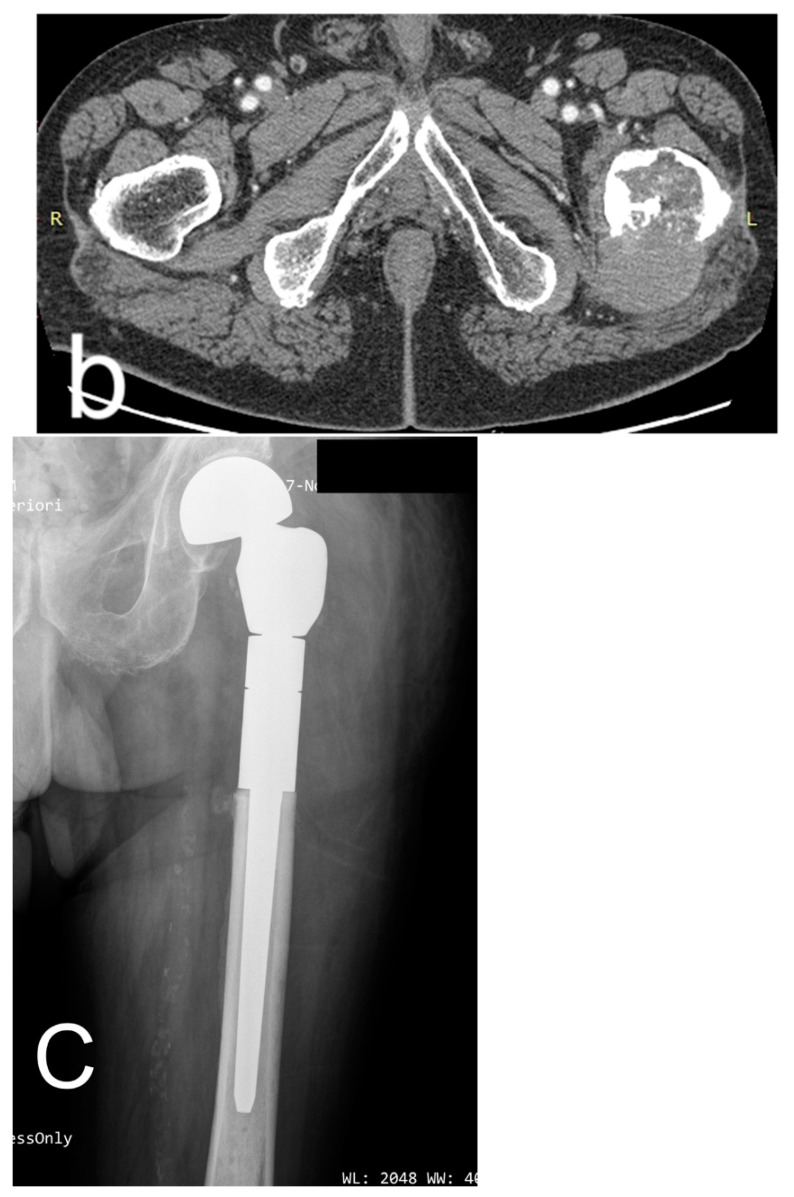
(a) Male, 84 years old, affected by dedifferentiated chondrosarcoma of the left proximal femur; CT coronal reconstruction of the proximal femur. (b) Axial CT scan shows extraosseous extension of the tumor. (c) Resection and reconstruction with a cemented proximal femur megaprosthesis were performed. The patient lived for 10 years (122 months) after surgery with no tumor recurrence and was able to walk with the aid of a cane. He died at 122 months due to a concomitant disease.
